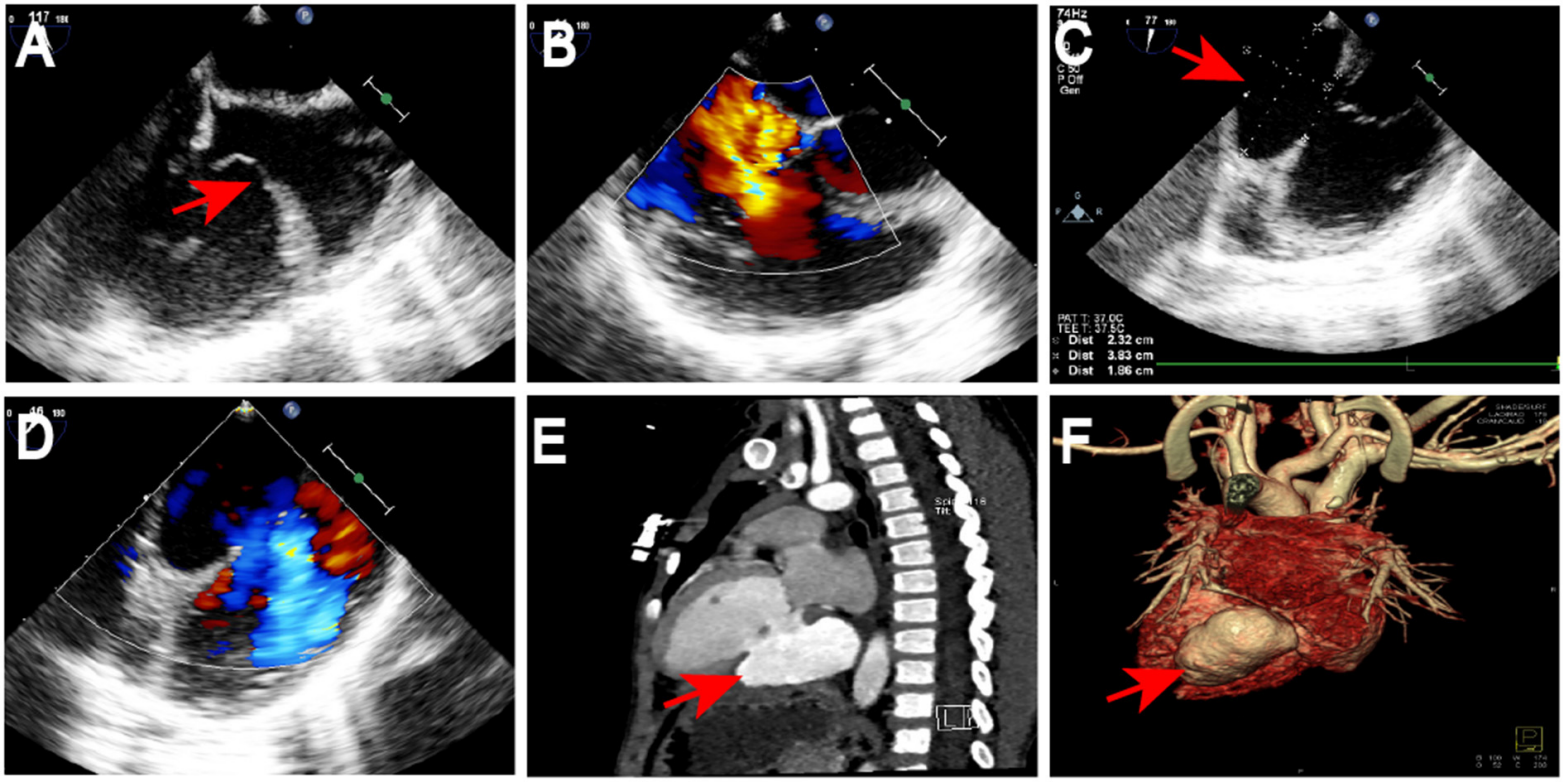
Preoperative echocardiography and computed tomography results. Anterior tricuspid leaflet prolapse was observed by TTE (red arrow; A). Tricuspid prolapse resulted in moderate tricuspid regurgitation (B). TTE revealed avulsion of anterior tricuspid leaflet resulting in moderate tricuspid regurgitation, a 23 mm × 38 mm giant aneurysm with a 19-mm diameter of the neck in the LVPW (red arrow; C) and visible blood flow into the aneurysm (D). CT scan and reconstruction confirmed a massive ventricular aneurysm in the LVPW (red arrow; E,F). TTE, Transthoracic Echocardiography; LVPW, left ventricular posterior wall; CT, computed tomography.
